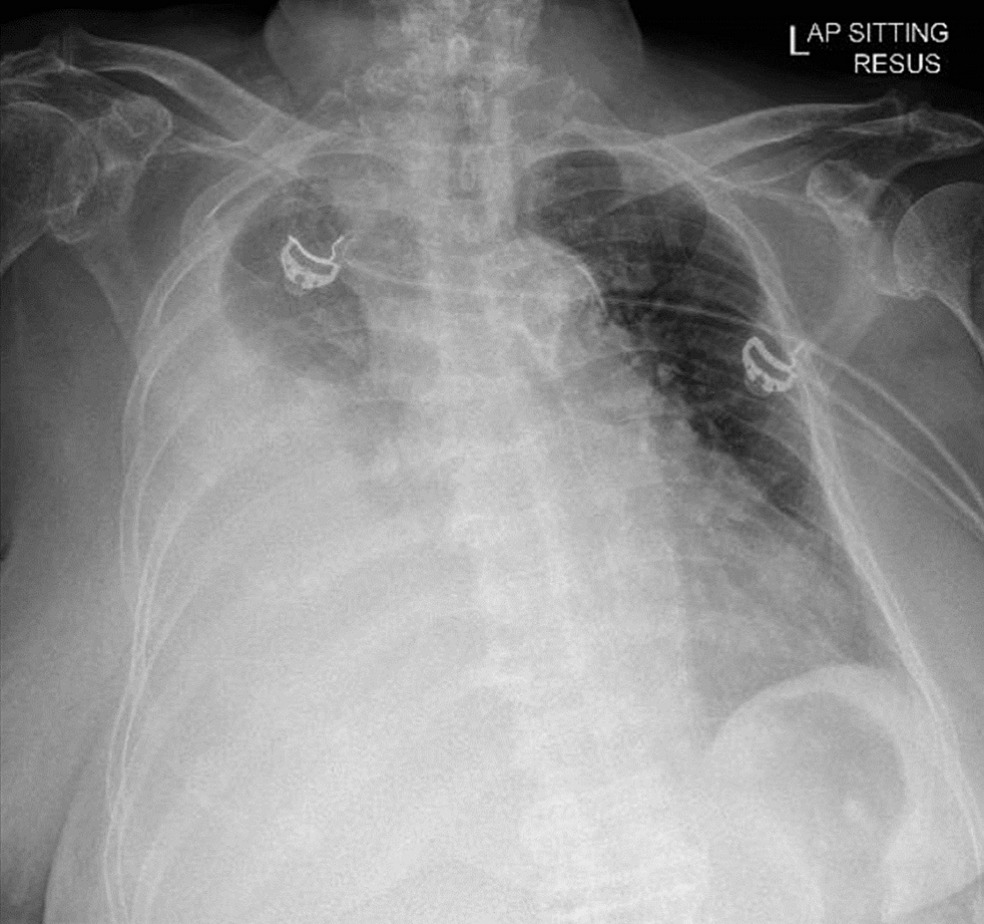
Chest radiograph revealing a large right pleural effusion.
